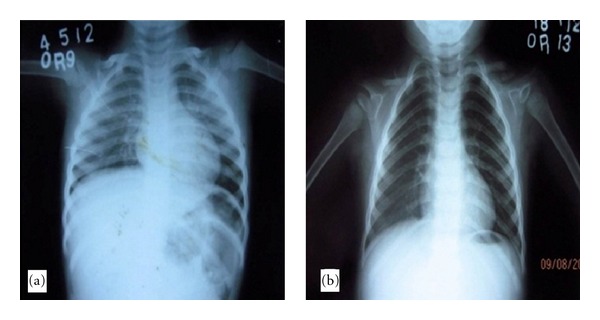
Chest radiograph: (a) patient and (b) sister.
